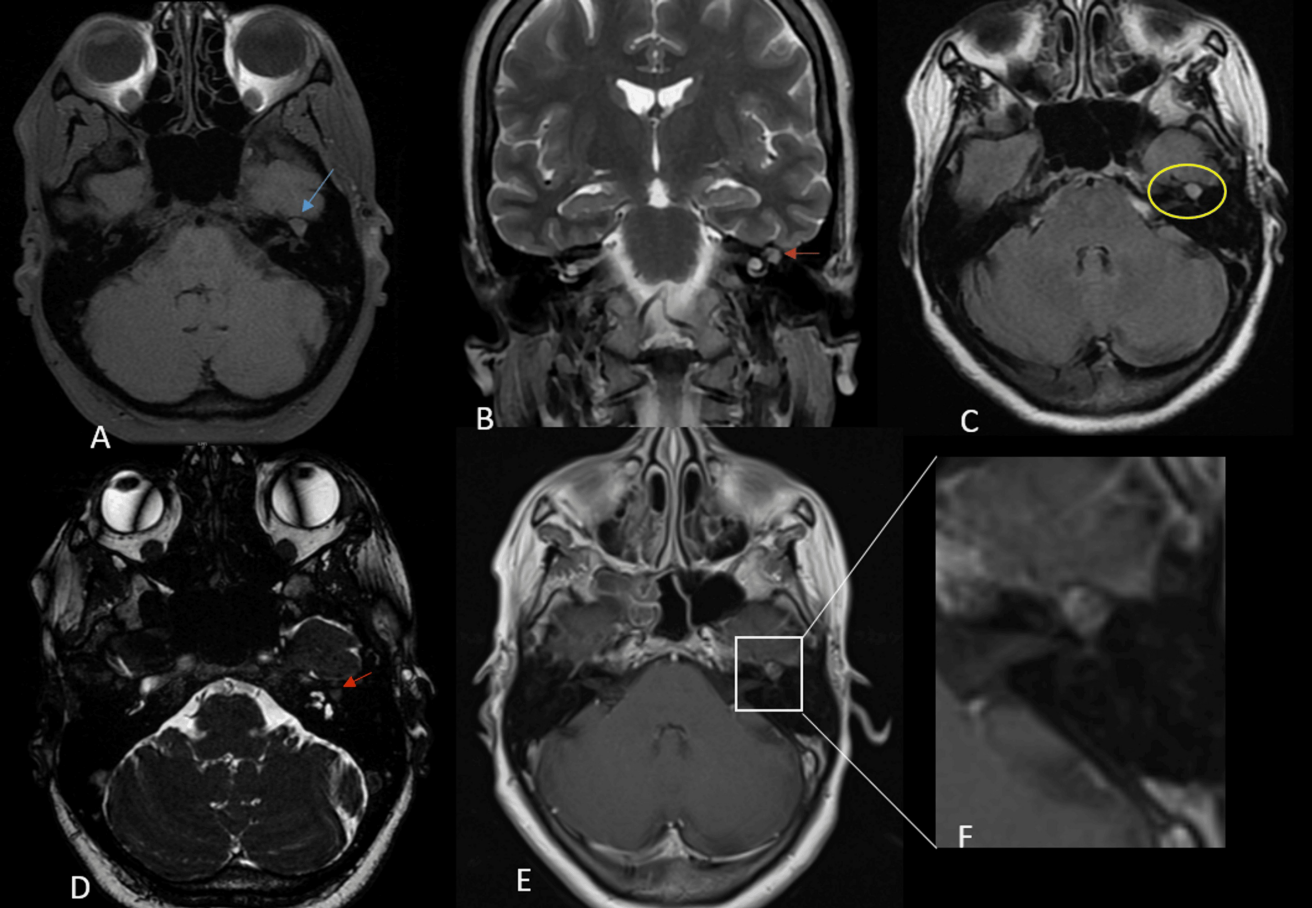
Brain MRI findings. Axial T1-weighted image (A), coronal T2-weighted image (B), axial fluid-attenuated inversion recovery (FLAIR) image (C), axial 3D fast imaging employing steady-state acquisition (FIESTA) sequence (D), and post-contrast axial T1-weighted image (E) with a zoomed-in view centered on the temporal lesion (F). MRI demonstrates a small nodular lesion along the intratemporal course of the left facial nerve, centered at the geniculate ganglion. The lesion is isointense on T1-weighted images (blue arrow), heterogeneously hyperintense on T2-weighted images with punctate hypointense foci (orange arrow), with slight hyperintensity on the 3D FIESTA sequence (red arrow). Post-gadolinium images demonstrate heterogeneous enhancement with punctate non-enhancing areas (white rectangle).
